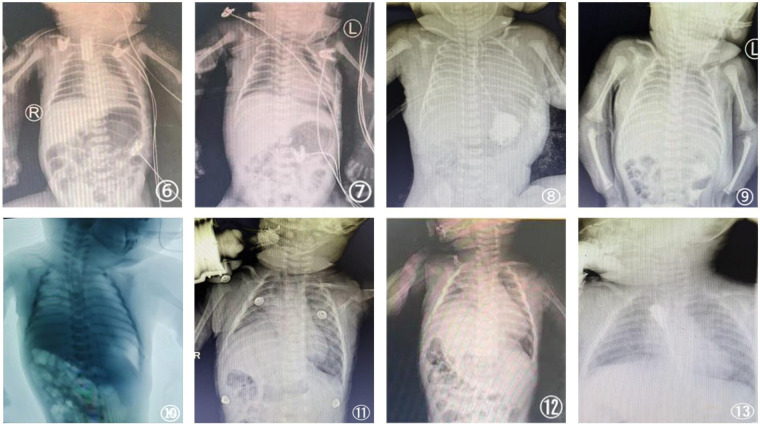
Serial chest imaging studies of case 2 (diaphragmatic plication). (6) (Preoperative): Esophagogram showing the proximal esophageal pouch with normal right hemidiaphragm position. (7) (Postoperative day 3): Bedside chest x-ray showing normal right hemidiaphragm anatomy. (8) (Postoperative day 10): Bedside chest x-ray showing bilateral lung consolidation (“white lung” phenomenon). (9) (Postoperative day 14): Bedside chest x-ray revealing new elevation of the right hemidiaphragm. (10) Fluoroscopy image demonstrating paradoxical movement of the elevated right hemidiaphragm. (11) (1-month post-discharge): Chest x-ray showing persistent diaphragmatic elevation. (12) (2-month post-discharge): Chest x-ray confirming ongoing diaphragmatic elevation prior to plication. (13) (2-month post-plication): Chest x-ray showing the restored normal anatomical position of the right hemidiaphragm.
